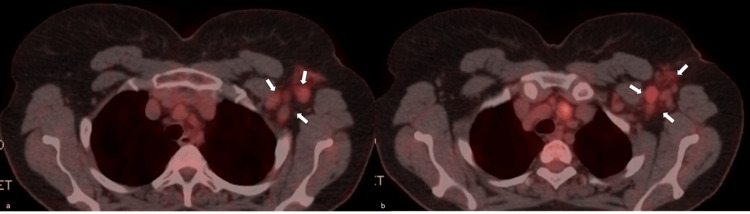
Positron emission tomography-computed tomography (PET-CT). The axial (a-b) PET-CT images show axillary lymph nodes (arrows) on the left axilla that demonstrate increased uptake of 18F-FDG (SUVmax = 3.4). Mediastinal blood pool SUV = 2.6. SUVmax: maximum standardized uptake value; SUV: standardized uptake value; 18F-FDG: 18F-fluorodeoxyglucose.
